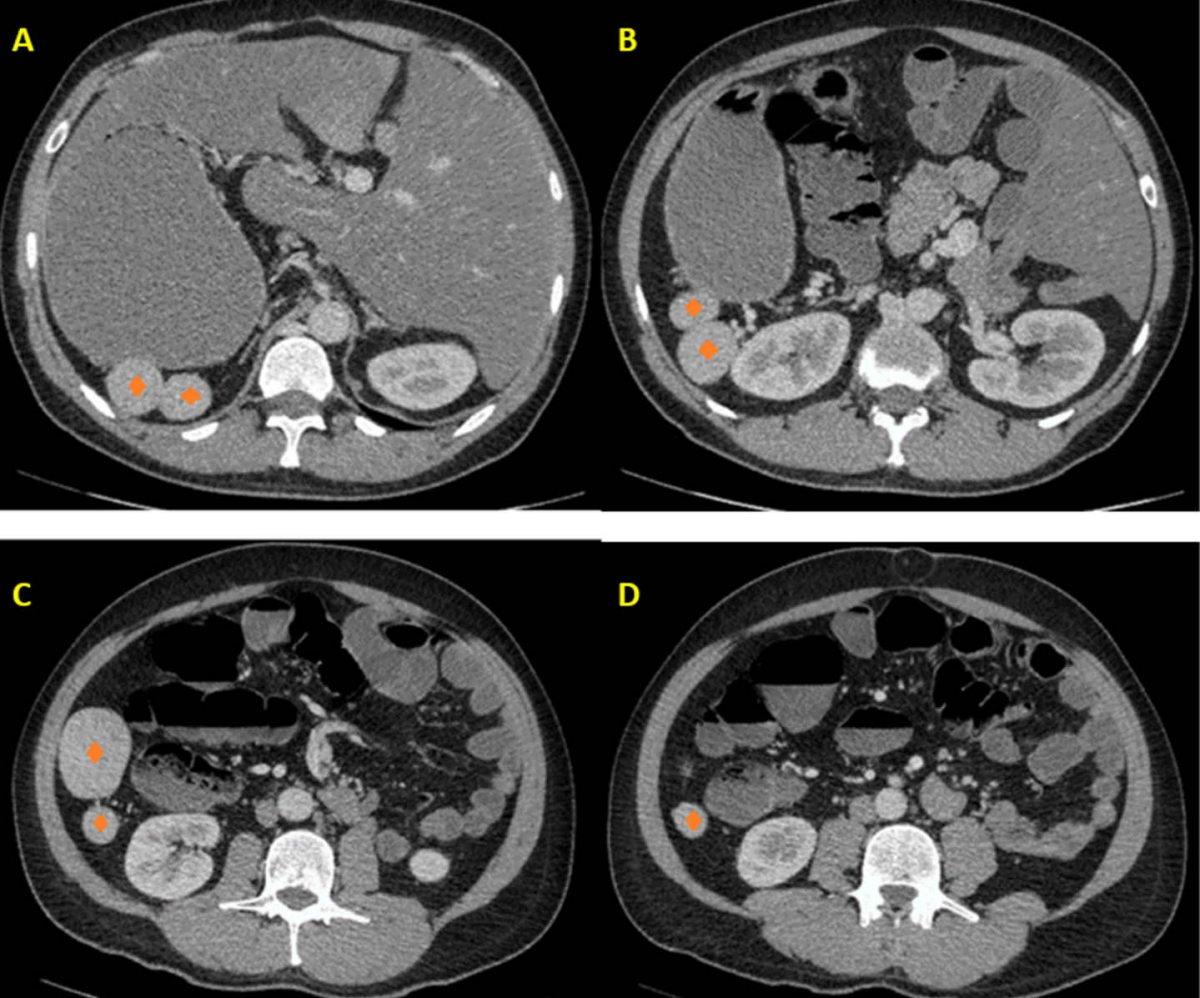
(A-D) Axial computed tomography craniocaudal sequential sections show multiple, well-circumscribed, soft tissue density nodular splenules (orange diamonds) located to the right of the abdomen with the ipsilaterally located stomach.
